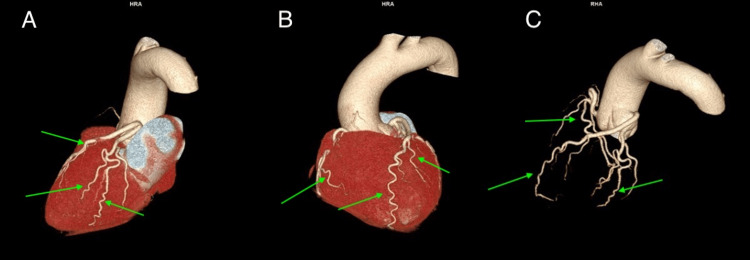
3D reconstruction of the coronary CTA (A) Significant tortuosity of multiple anterior coronary arteries (green arrows). (B) Significant tortuosity of multiple posterior coronary arteries (green arrows). (C) Heart removed, demonstrating the degree of tortuosity of several coronary arteries (green arrows). 3D: three dimensional, CTA: computed tomography angiography
